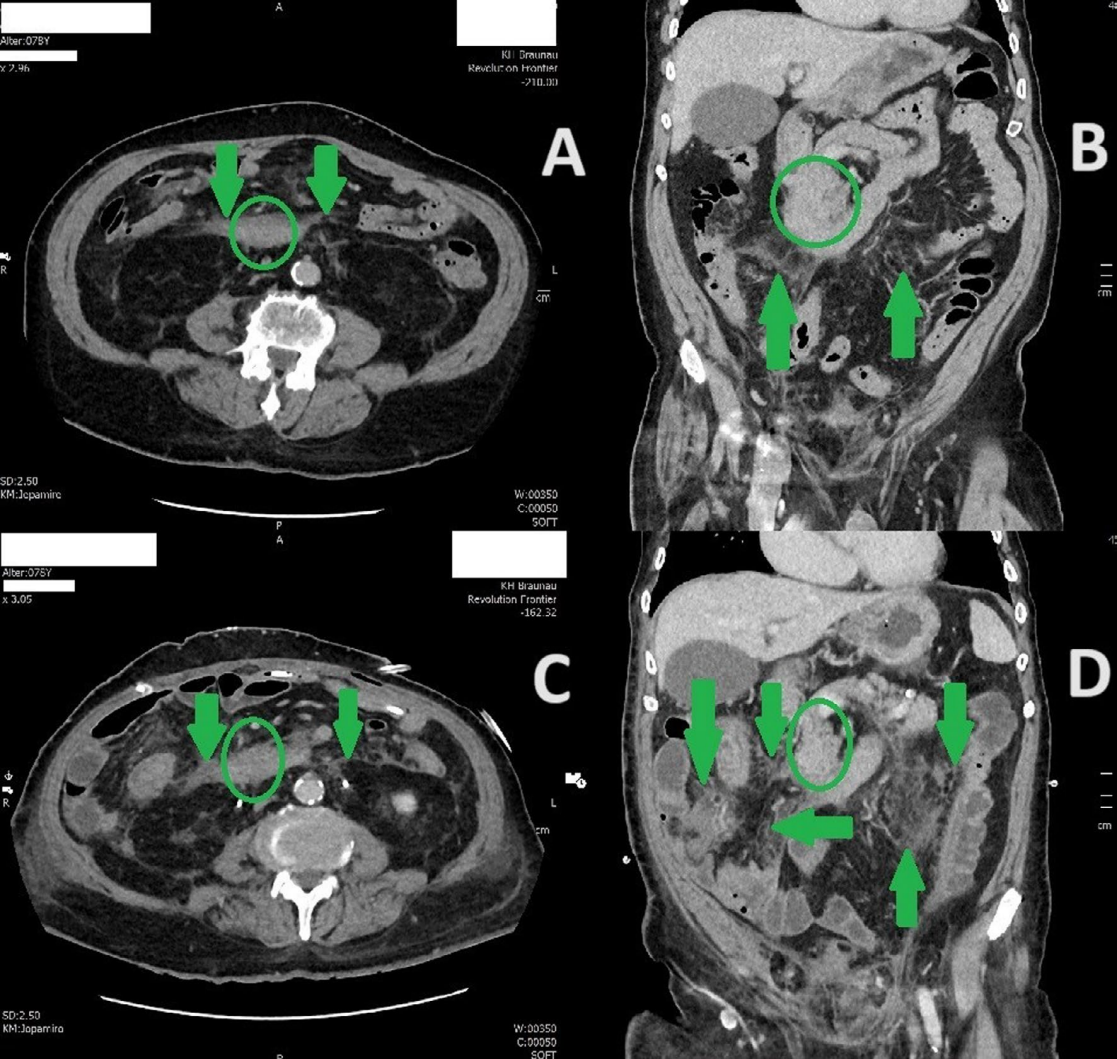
(A, B) First CT abdomen with contrast in axial and coronal plane showing hyperdense structure surrounding duodenum (encircled area) with lateral retroperitoneal spreading (green arrows). (C, D) Last CT scan before chemotherapy showing progression of the finding‐ in the meantime the lesion was proved to be peritoneal carcinomatosis.
